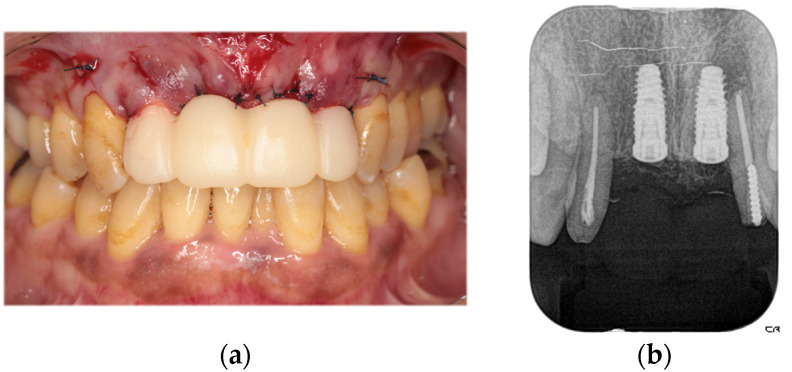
Intraoral photograph (a) and dental radiograph (b) immediately after implant placement.
